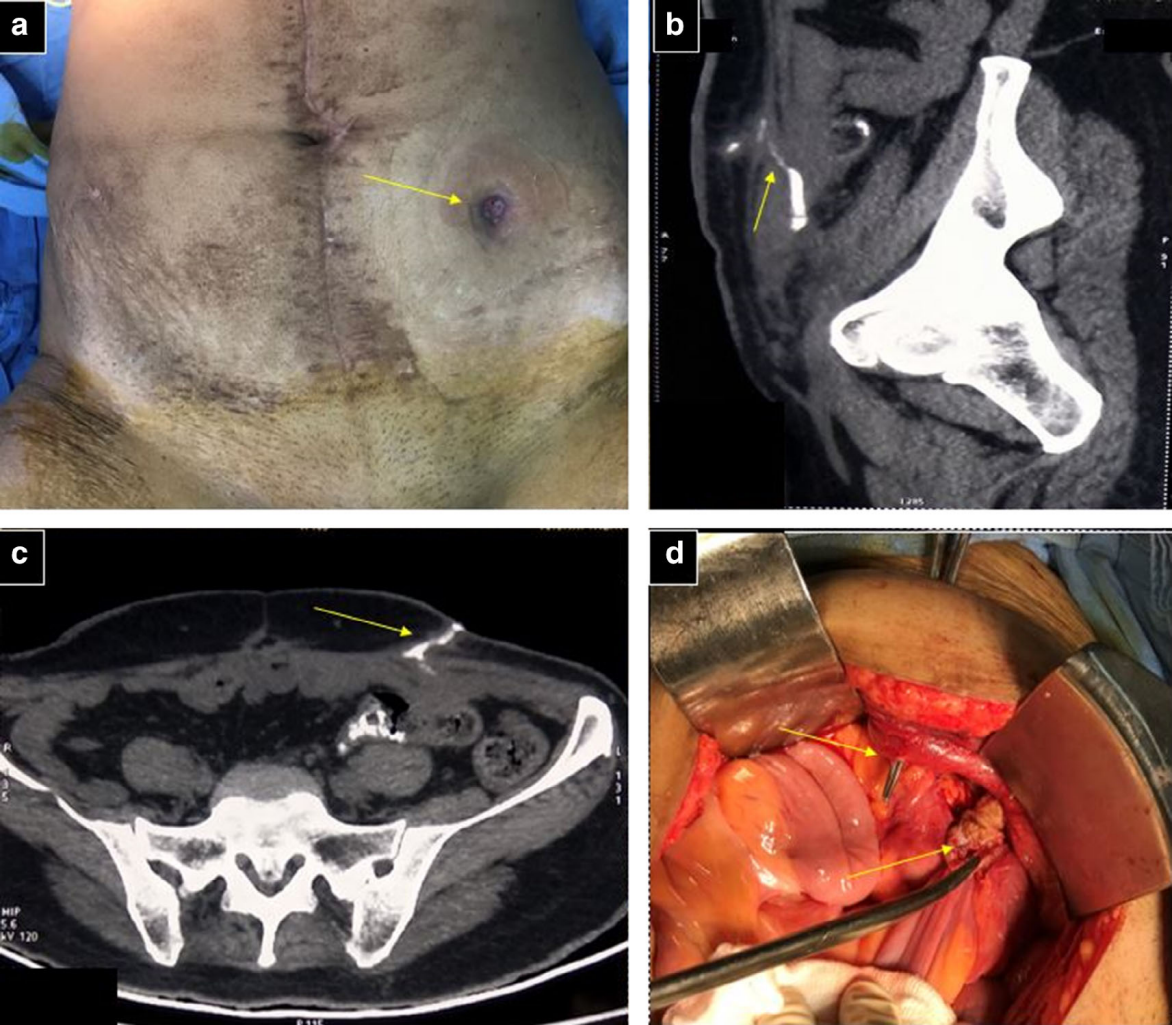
(a) Fistulous tract opening in left iliac fossa (yellow arrow). Computed tomography fistulogram shows thick‐walled communicating tract from the sigmoid colon to exterior (yellow arrow) in sagittal (b) and axial (c) views. (d) The intraoperative demonstration of enterocutaneous fistula (yellow arrow).
